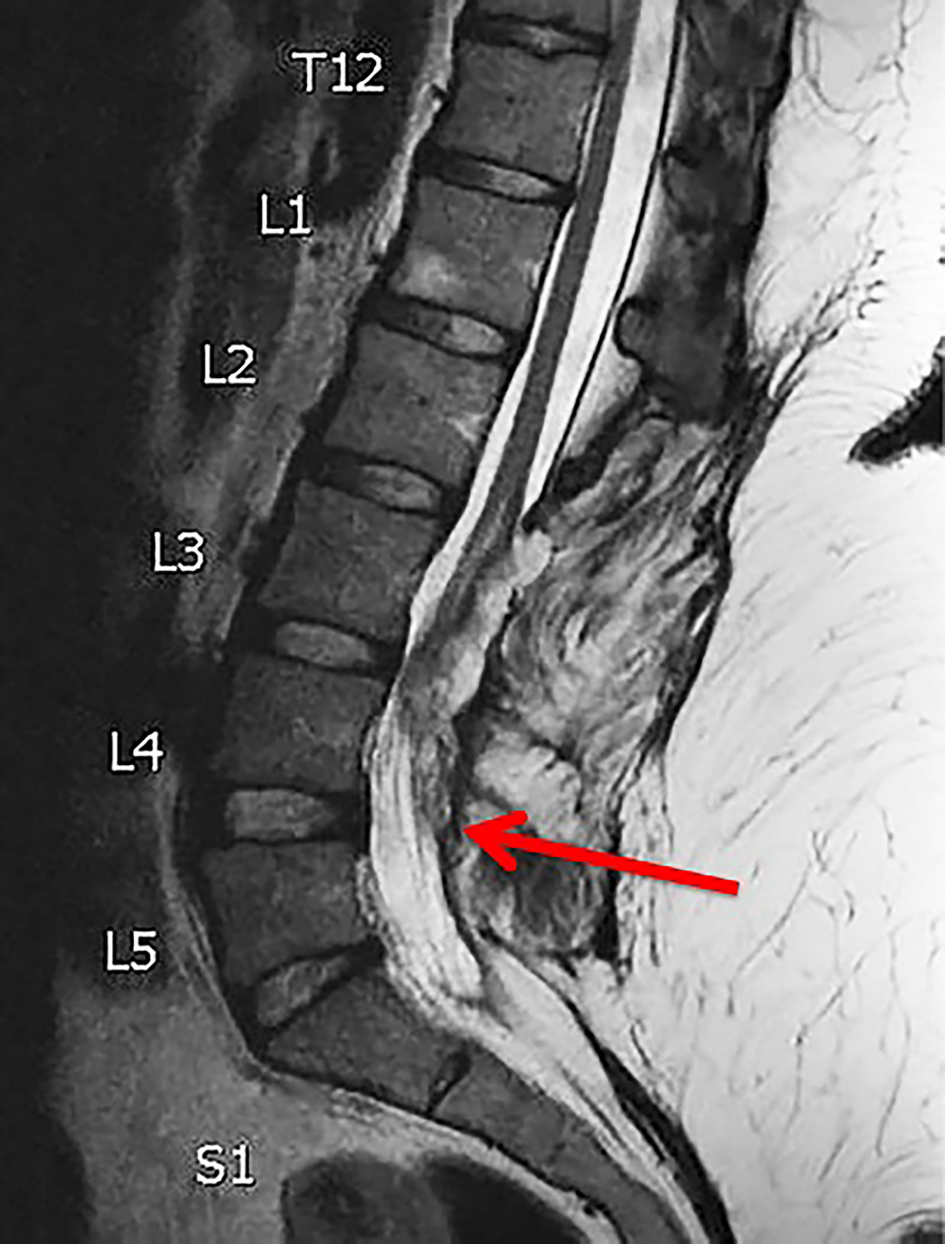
Preoperative MRI Sagittal T2 magnetic resonance imaging (MRI) demonstrating recurrent spinal cord tethering (demarcated by the red arrow)
